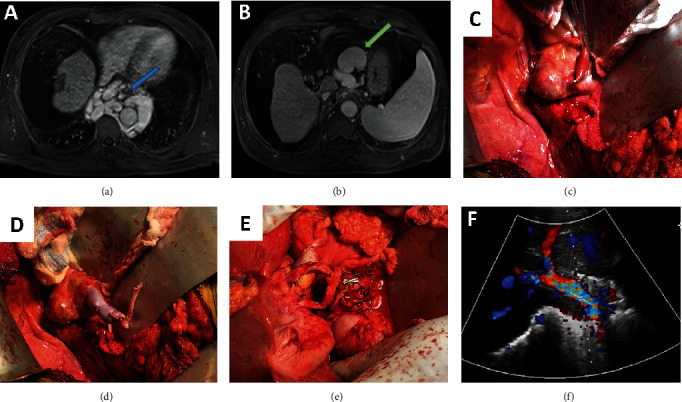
MRI, intraoperative, and ultrasound imaging demonstrating (a) extensive esophageal varices (blue arrow), (b) a large perigastric varix used for portal inflow (green arrow), (c) the clamped varix, (d) an ileac vein graft anastomosed to the varix, (e) the porta postimplantation with varicoportal inflow and the overlying hepatic artery, and (f) Doppler imaging of the varicoportal inflow.
